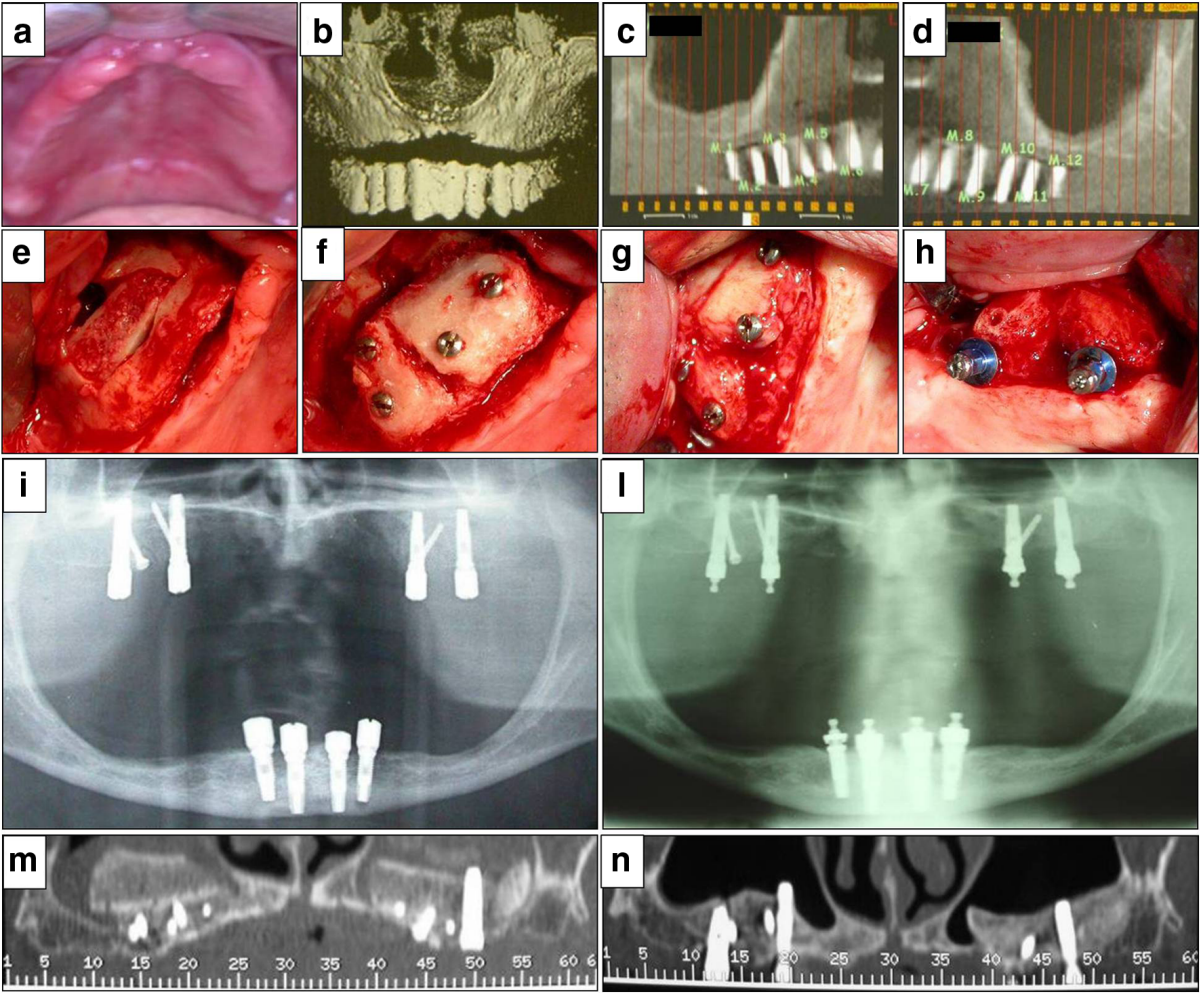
A standard case of total maxillary atrophy. Pre-operative images (a–d). Right side: inlay homograft (e), with cortical bones in its upper and lower parts; bone horizontal augmentation (f). Graft maturation after 6 months (g) and implant insertion (h). Post-operative images after 6 months (i) and 3 years (l). Comparable CT scans: an immediate post-operative view (m) and after 3 years (n)
